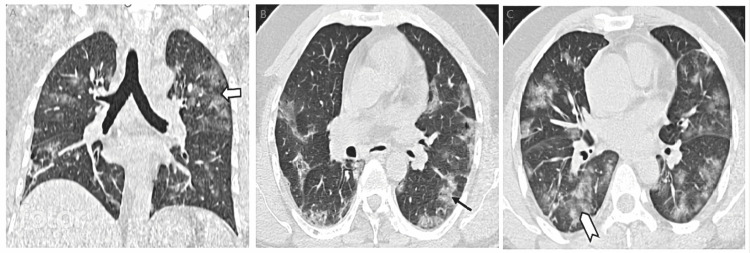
HRCT images of patients classified in moderate category. A: Coronal image showing GGOs involving bilateral lung parenchyma (moderate category). Block arrow showing areas of GGOs. B: Axial image showing GGOs involving bilateral lung parenchyma (moderate category). Arrow showing areas of GGOs with surrounding fibrotic changes. C: Axial image showing GGOs with surrounding consolidatory changes (moderate category). Chevron shows areas of GGOs and consolidatory changes. GGOs, ground glass opacities
